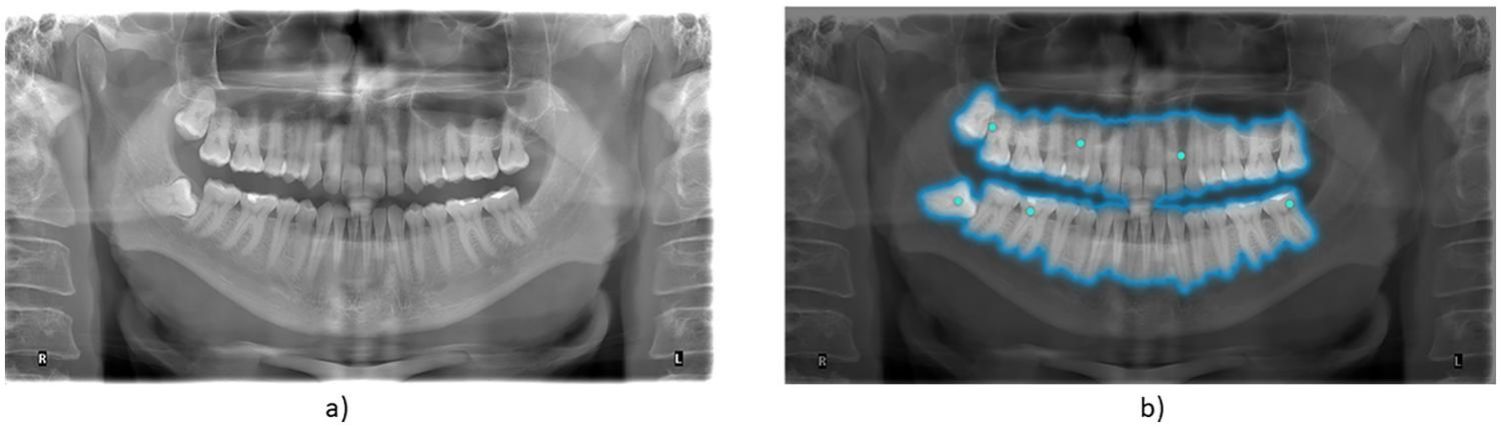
An example orthopantomogram (OPG) shown without (a) and with (b) teeth partitioning by AI segmentation (SAM2 algorithm). The segmentation in Figure 1b was obtained with tools available at https://github.com/facebookresearch/segment-anything, licensed under Apache License, Version 2.0.
